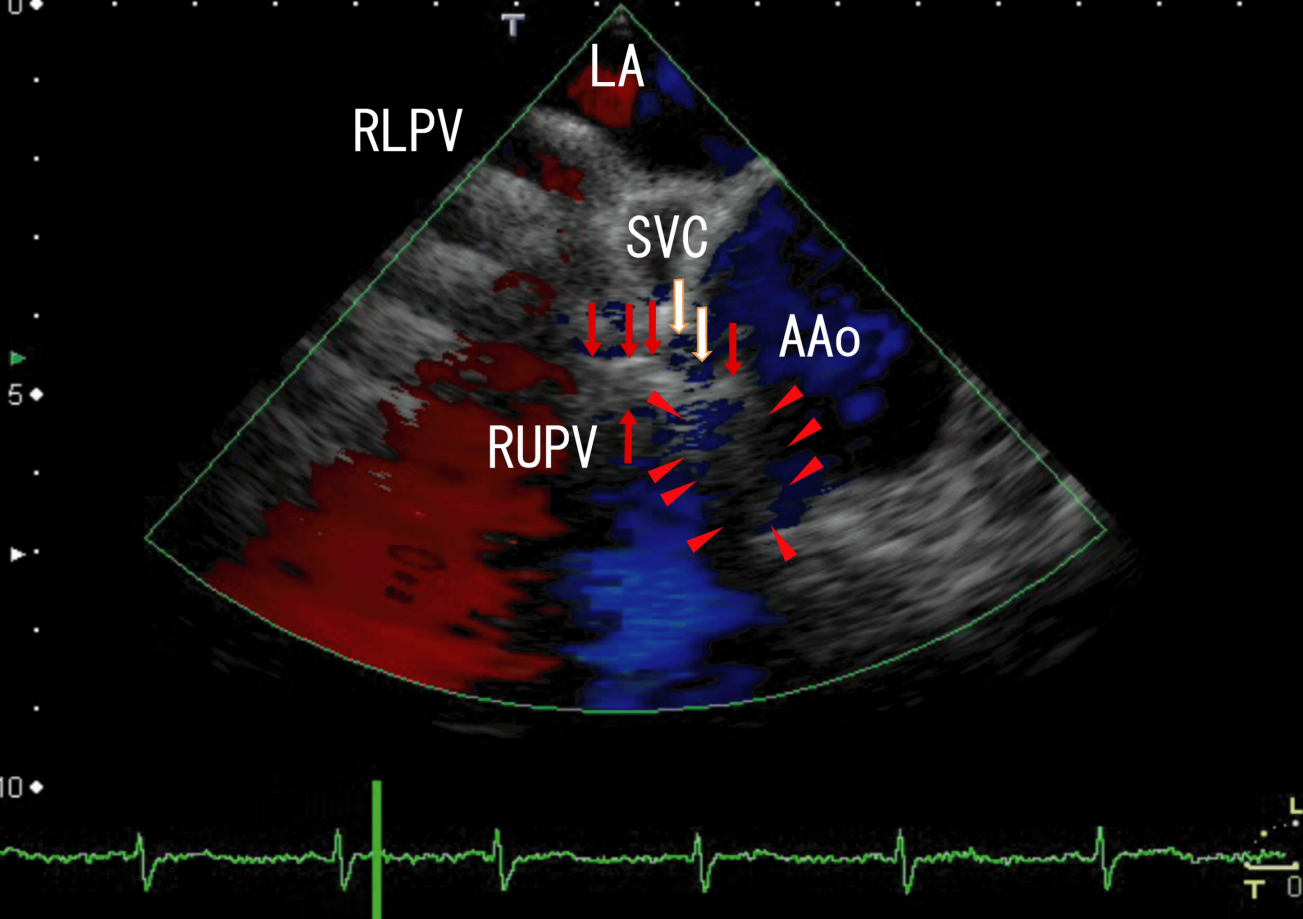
Transesophageal echocardiography (TEE) images showing ascending aorta (AAo) thrombi and linear white thrombi The TEE revealed thrombi in the AAo and linear white thrombi from the RUPV into the AAo (red arrows), and the linear white thrombi were situated in the anterior of the SVC. There were small blue areas between the end of the AAo wall and the linear white thrombi on the AAo wall (white arrows), indicating a cleft in the AAo wall and penetration of the AAo wall by the thrombi. Approximately 2 cm of the right anterior AAo wall appeared to have lost its normal structure and formed a dark-white mass (arrowheads). The red areas in the LA indicate blood flow from the RUPV. AAo: Ascending aorta; LA: Left atrium; RLPV: Right lower pulmonary vein; RUPV: Right upper pulmonary vein; SVC: Superior vena cava.
